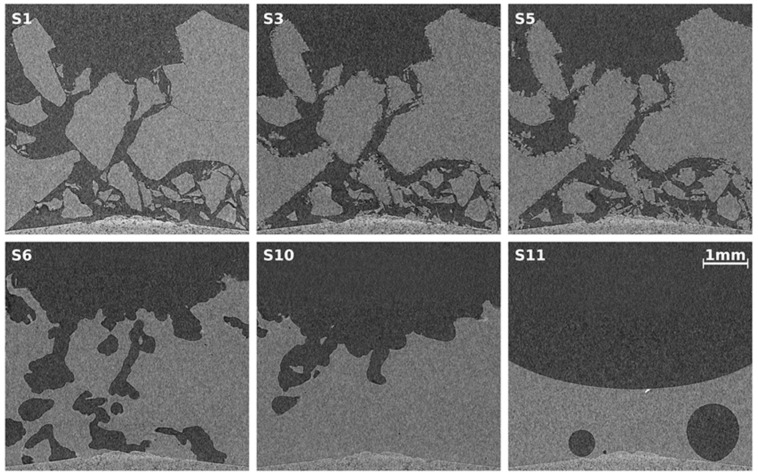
Tomographic images obtained from CT scans (numbered S1, S3, S5, S6, S10, and S11) of the THF clathrate sample at different points in time. Each slice represents the exact same position in space. In S1–S5 the sample temperature is 247 K, in S6 it is 273 K, and in S10 it is 274 K. S11 shows the meniscus of the molten sample together with gas bubbles, which can be found all over the liquefied sample. The bright point in slice S11 stems from a tiny metallic particle.
